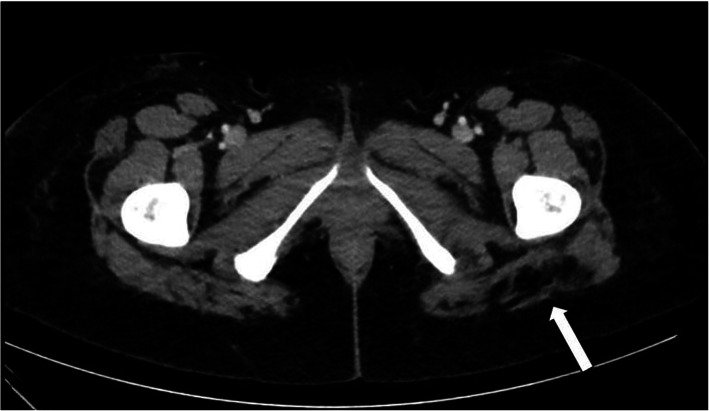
Computed tomography (CT) image of the lower gluteal region of the patient in case 2. White-colored areas correspond to the bones. The white arrow indicates areas of low attenuation (black) corresponding to significant fat within the inferior aspect of the left Gluteus Maximus muscle adjacent to the sciatic nerve (SN)
